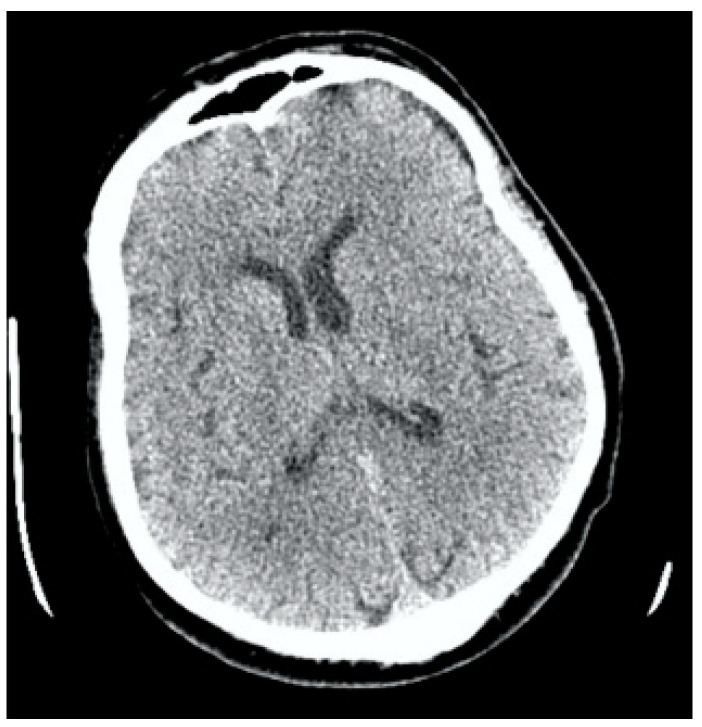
Clinical Case 2, Patient S., male, 60 years old. Cerebral MSCT in 30 min after the onset of symptoms. There are no data for ischemia and brain damage, ASPECTS = 10.
